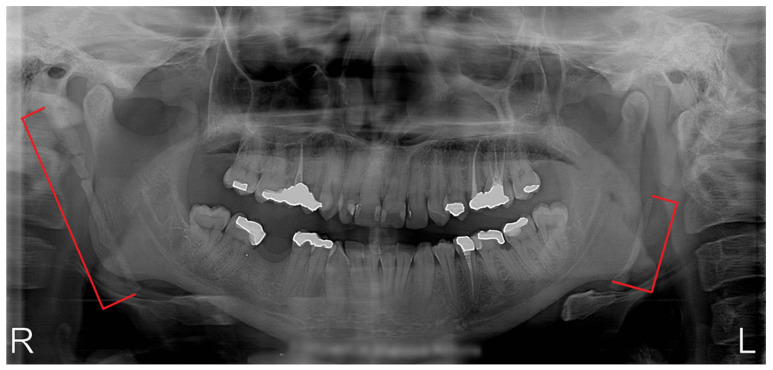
Panoramic radiograph of a patient with bilateral elongation of the styloid process.
